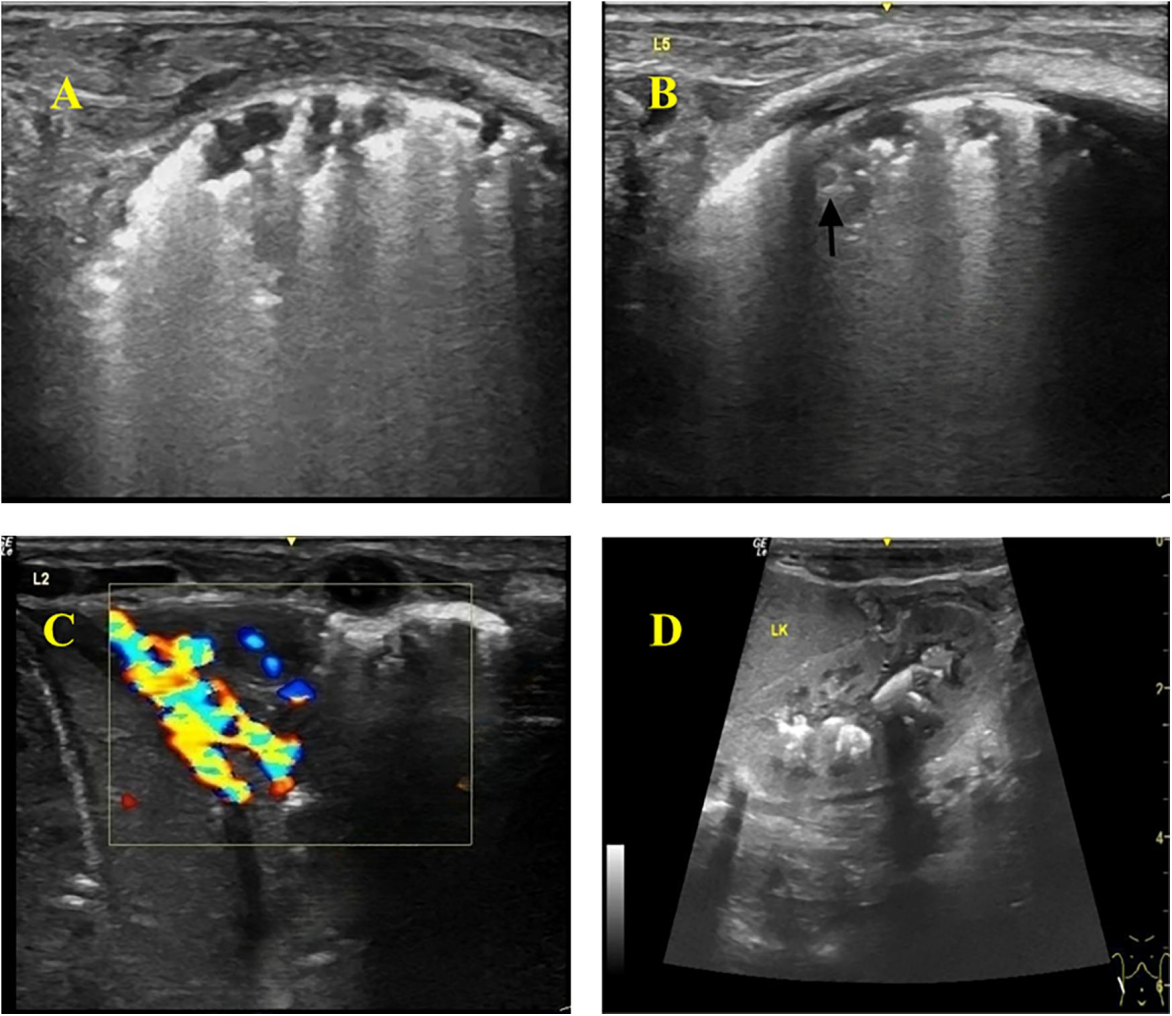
Color Doppler ultrasonography of the infant. (A) Thickened and hyperechoic pleural lines in both lungs, with partial discontinuity. (B) Multiple discontinuous small subpleural hypoechoic lesions (*ragged cloth sign*) with scattered hyperechoic patchy areas (*fragmentation sign*) at the margins. Nodular changes within some consolidated areas. (C) Wedge-shaped consolidation in the lower lobes of both lungs, with heterogeneous echogenicity and small hypoechoic areas, showing rich blood flow signals on Doppler. (D) Abdomen (Color Doppler Ultrasound): Hepatomegaly and splenomegaly. Calcifications visible in the renal pelvises and calyces of both kidneys.
